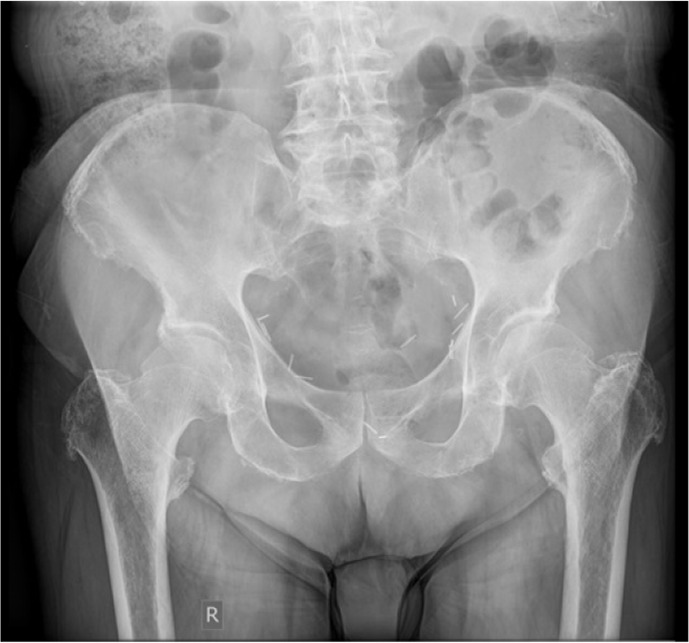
Pelvis AP radiograph. There is whiskering enthesopathy more pronounced in the greater and lesser trochanters and more subtle in the iliac crests and the ischial tuberosities. The sacroiliac joints are fused to some extent and sclerotic.
